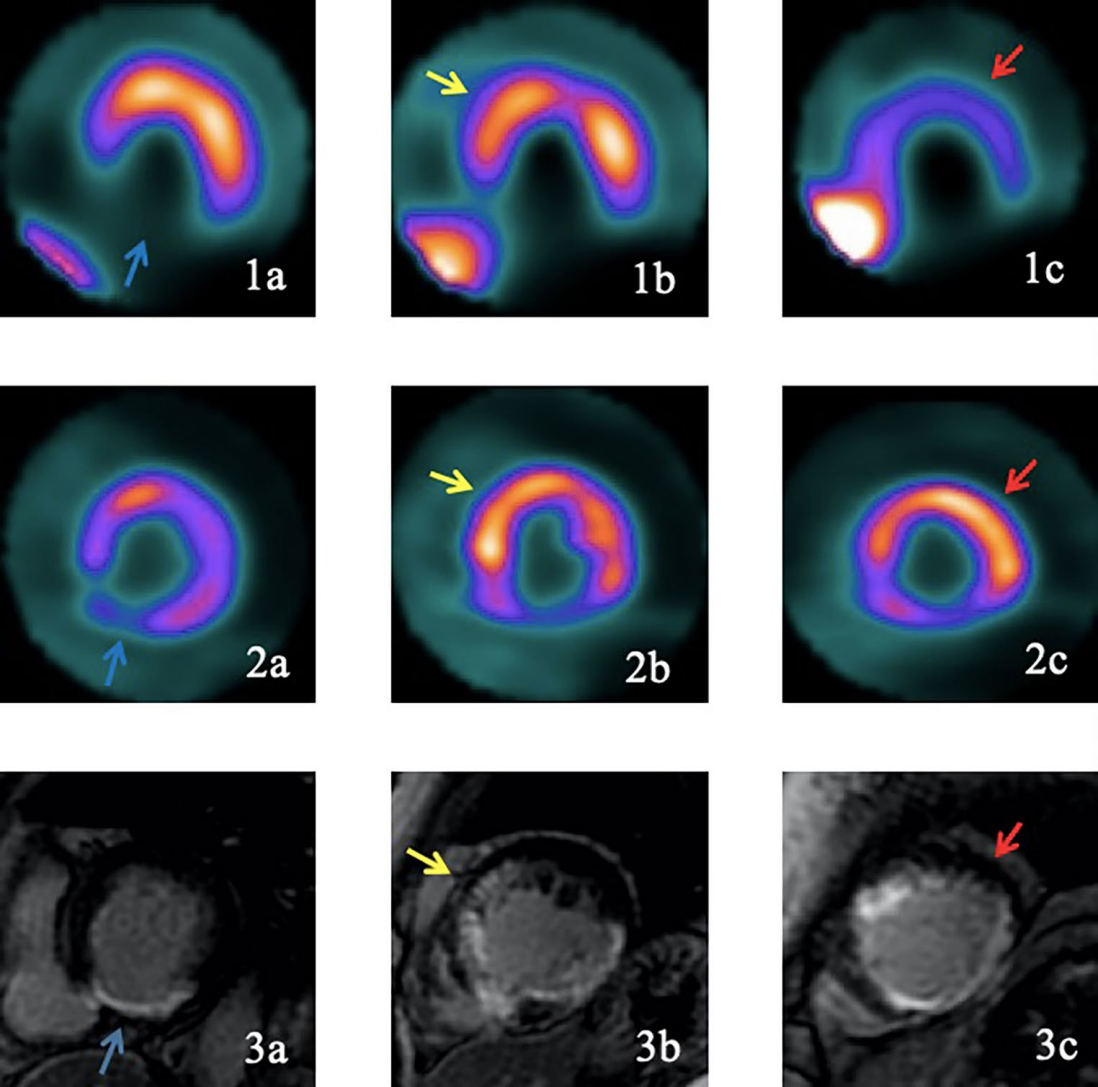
A 67-year-old male patient with coronary heart disease (double vessel disease), and the preoperative LVEF was 38%. The Fig. 2a and b, and 2c shows the ^13^N-NH_3_PET myocardial perfusion images; Fig. 3a and b, and 3c shows the ^18^F-FDG PET myocardial metabolic images; Fig. 1a and b, and 1c shows the DE-CMR images. All images showed short-axis view; a, left basal ventricle; b, left middle ventricle; and c, apex of the left ventricle. The PET myocardial perfusion and metabolism of lower wall of the basal left ventricle and part of the lower septal wall generally showed equally reduced uptake or no uptake (Figs. 2a and 3a). DE-CMR indicated transmural enhancement (Fig. 1a) (blue arrow). The perfusion and metabolism of the anterior septal wall of left ventricle were normal, indicating viable myocardium (Figs. 2b and 3b); while DE-CMR indicated transmural enhancement (Fig. 1b), and the findings of these two imaging methods were inconsistent (yellow arrows). The perfusion of anterior wall of the left ventricular apex was substantially reduced, while the metabolism was normal, and the perfusion/metabolism mismatch was present, indicating the presence of hibernating myocardium (Figs. 2c and 3c); DE-CMR indicated subendocardial enhancement (Fig. 1c) (Red arrow)
